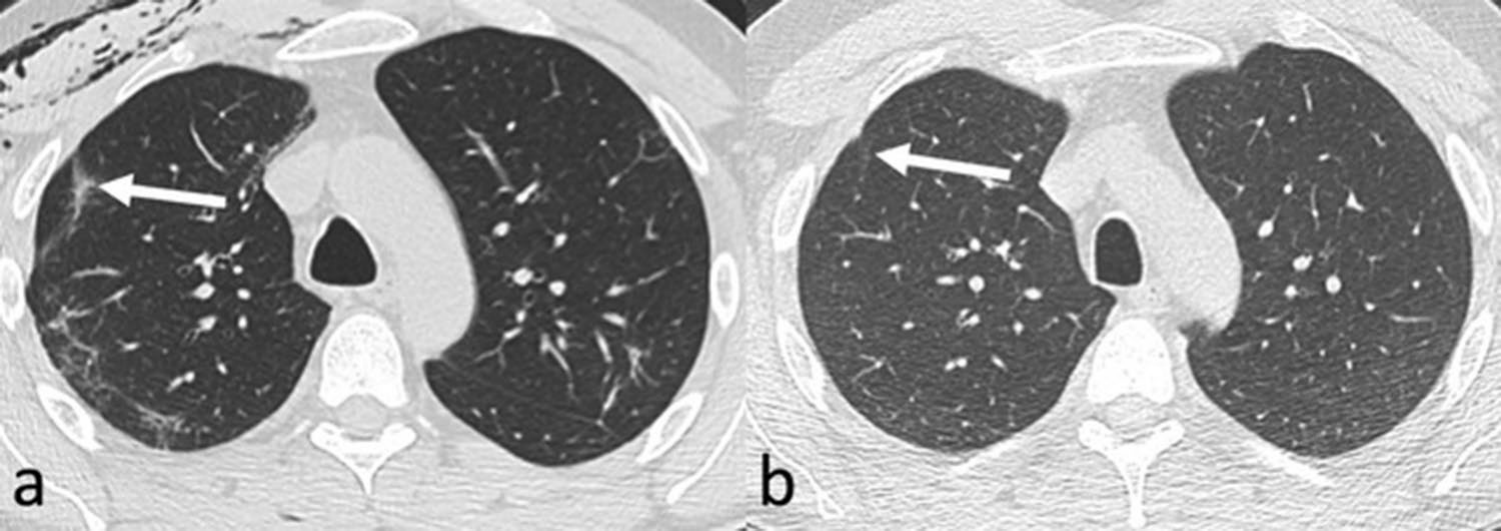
Radiological follow-up after tension pneumothorax due to COVID-19 pneumonia. Thoracic computed tomography performed 1 day before second discharge from the hospital (a) shows subpleural linear and reticular consolidations as a sign of COVID-19 pneumonia (arrow). Furthermore, there is right-sided soft tissue emphysema due to the pneumothorax (the latter not shown). One year later (b), the pulmonary abnormalities due to COVID-19 pneumonia have almost completely resolved. Only very few, discrete subpleural linear consolidations and GGO (arrow), which presumably correspond to minor post-inflammatory fibrotic changes, are visible. *COVID-19* coronavirus disease 2019, *CT* computed tomography, *GGO* ground-glass opacities
